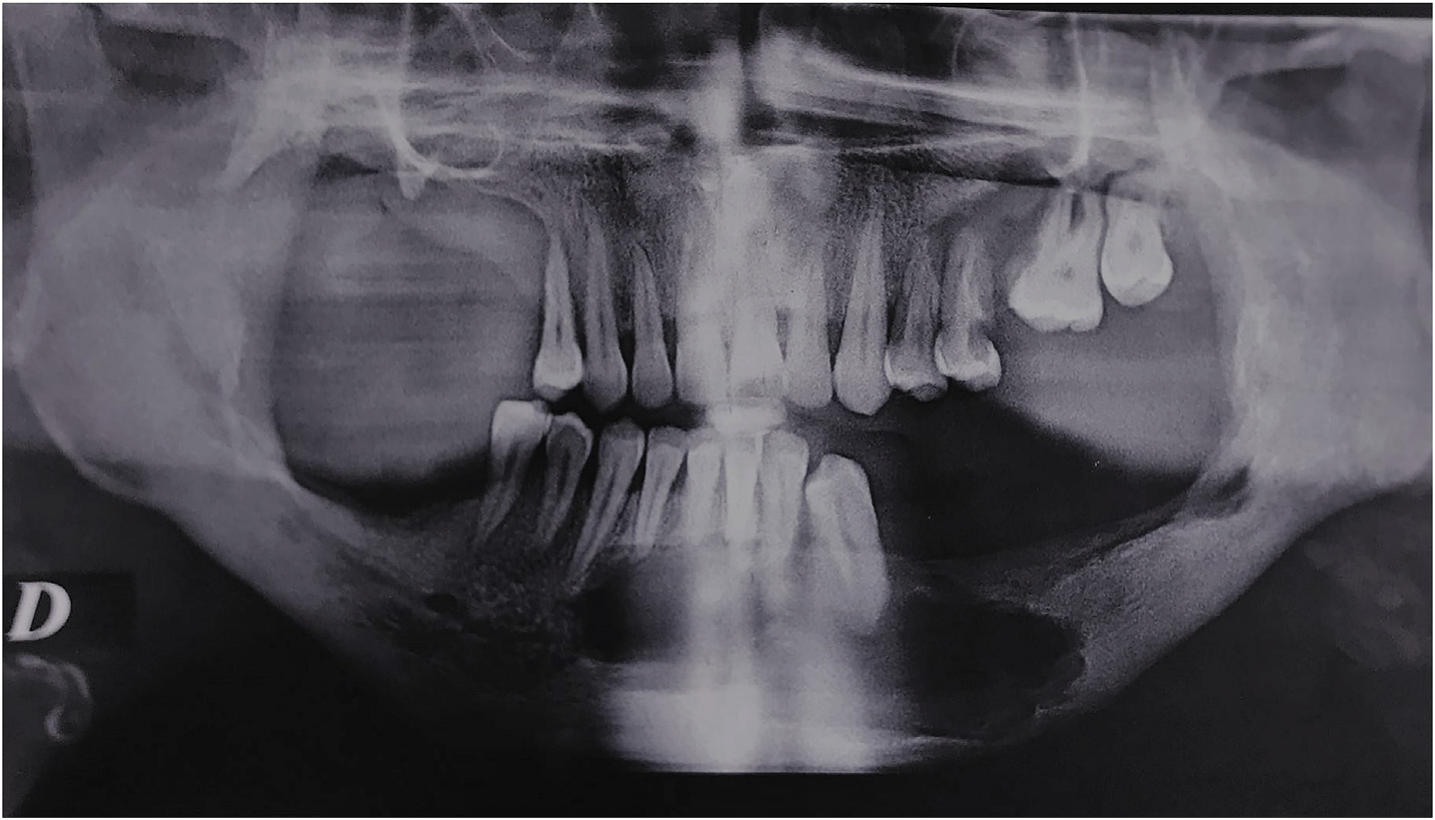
Pre-operative orthopantomogram showing bifocal cystic lesions (mandible and left maxilla) and multiple radiolucent areas in the alveolar ridge.
